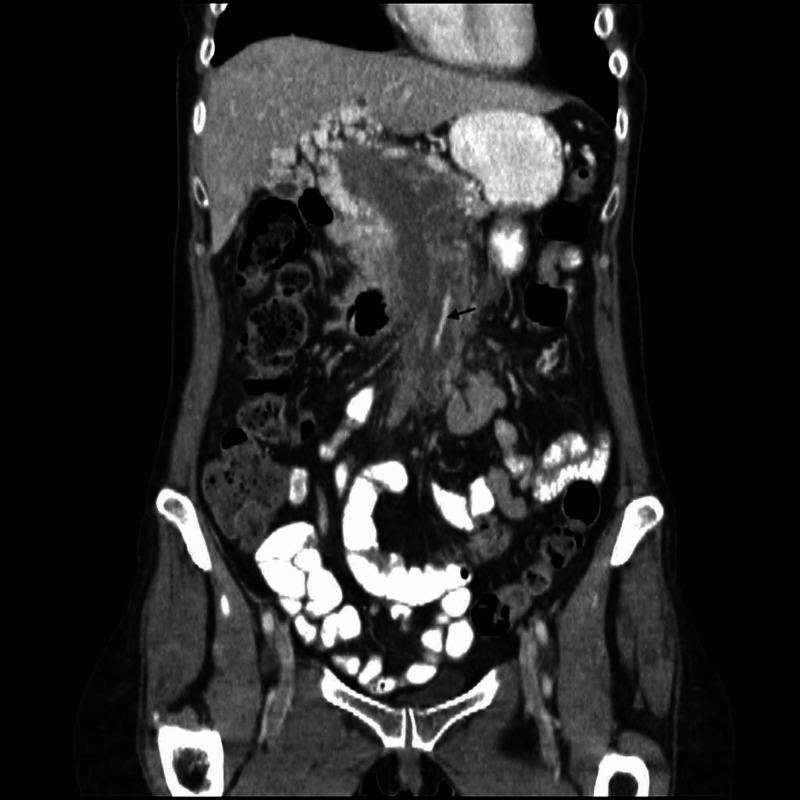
Coronal plane abdominal contrast-enhanced CT showing a large (7.2 cm x 2.5 cm) heterogeneous soft tissue mass within the root of the mesentery surrounding the superior mesenteric artery (black arrow).
